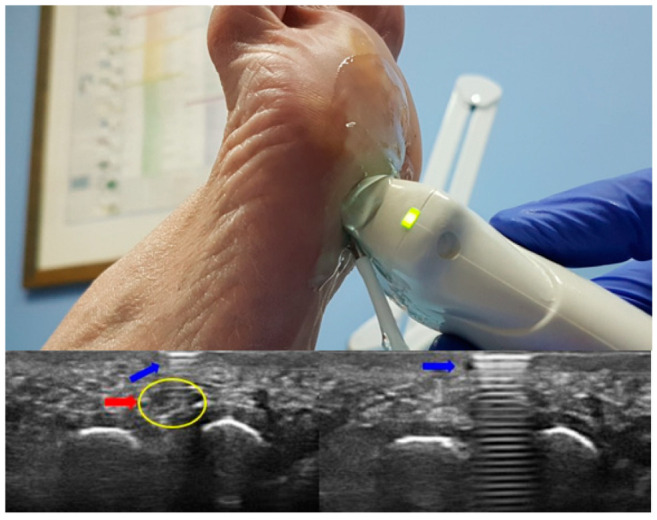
Painful echopalpation of MN: the tip of the pen presses on Morton’s neuroma, causing pain. The ultrasound image shows “the comet’s tail” produced by the tip of the pen. The blue arrow indicates the hyperechoic image produced by the pen, and the red arrow indicates the position of the MN. The yellow circle shows the MN.
